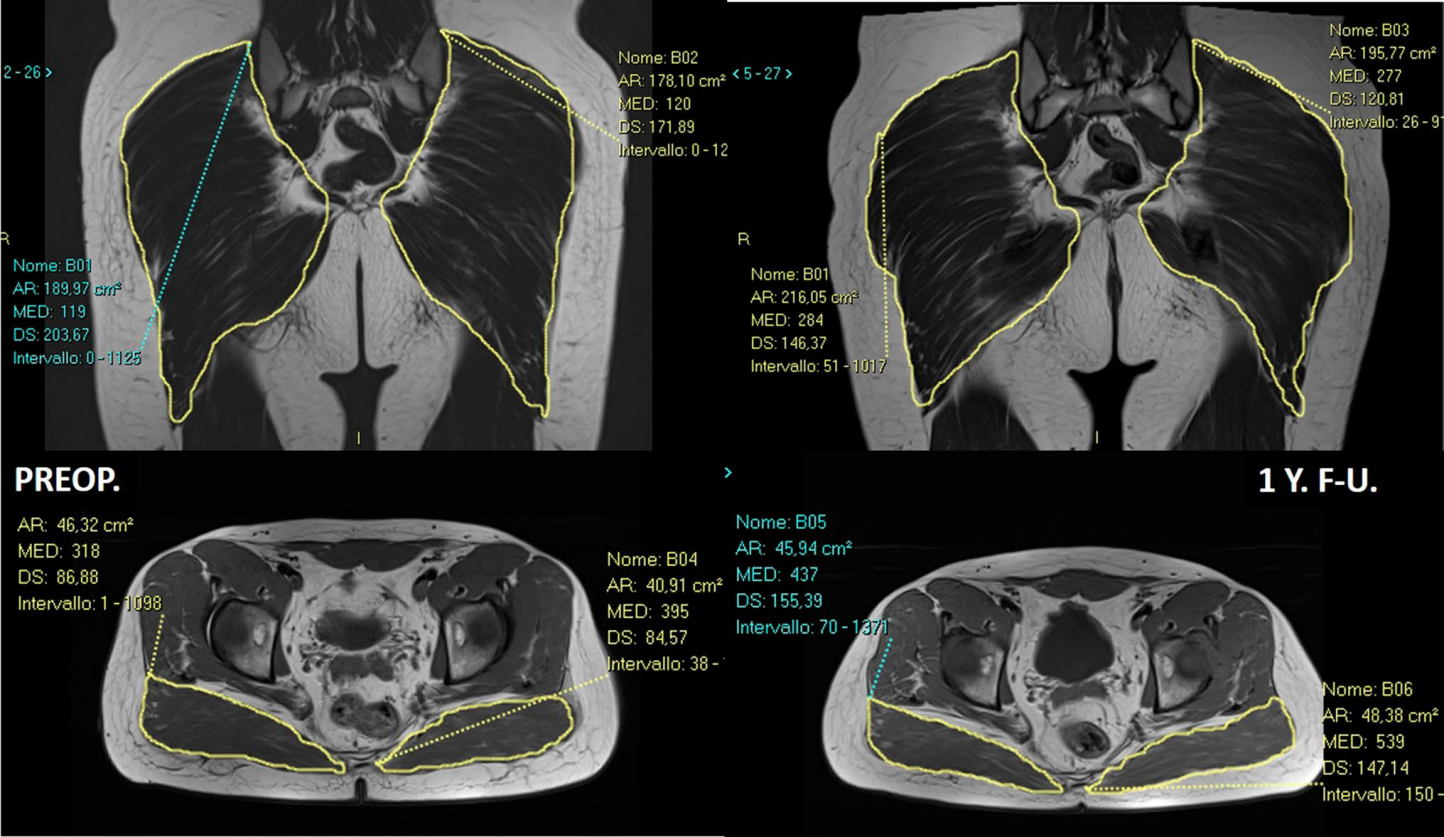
This is the MRI of a 31-year-old boy, who was operated for a femoro-acetabular impingement syndrome and external snapping hip syndrome of the right hip. Once the intra-articular procedures have been performed, the endoscopic release of the gluteus maximus tendon have been done. At 1-year follow-up, the patient was pain free, with no recurrence of the snap. The MRI shows that there are no significant differences in the cross-sectional area of the gluteus maximus muscle between the two sides, nor a significant fatty infiltration of the operated side at 1 year from surgery
